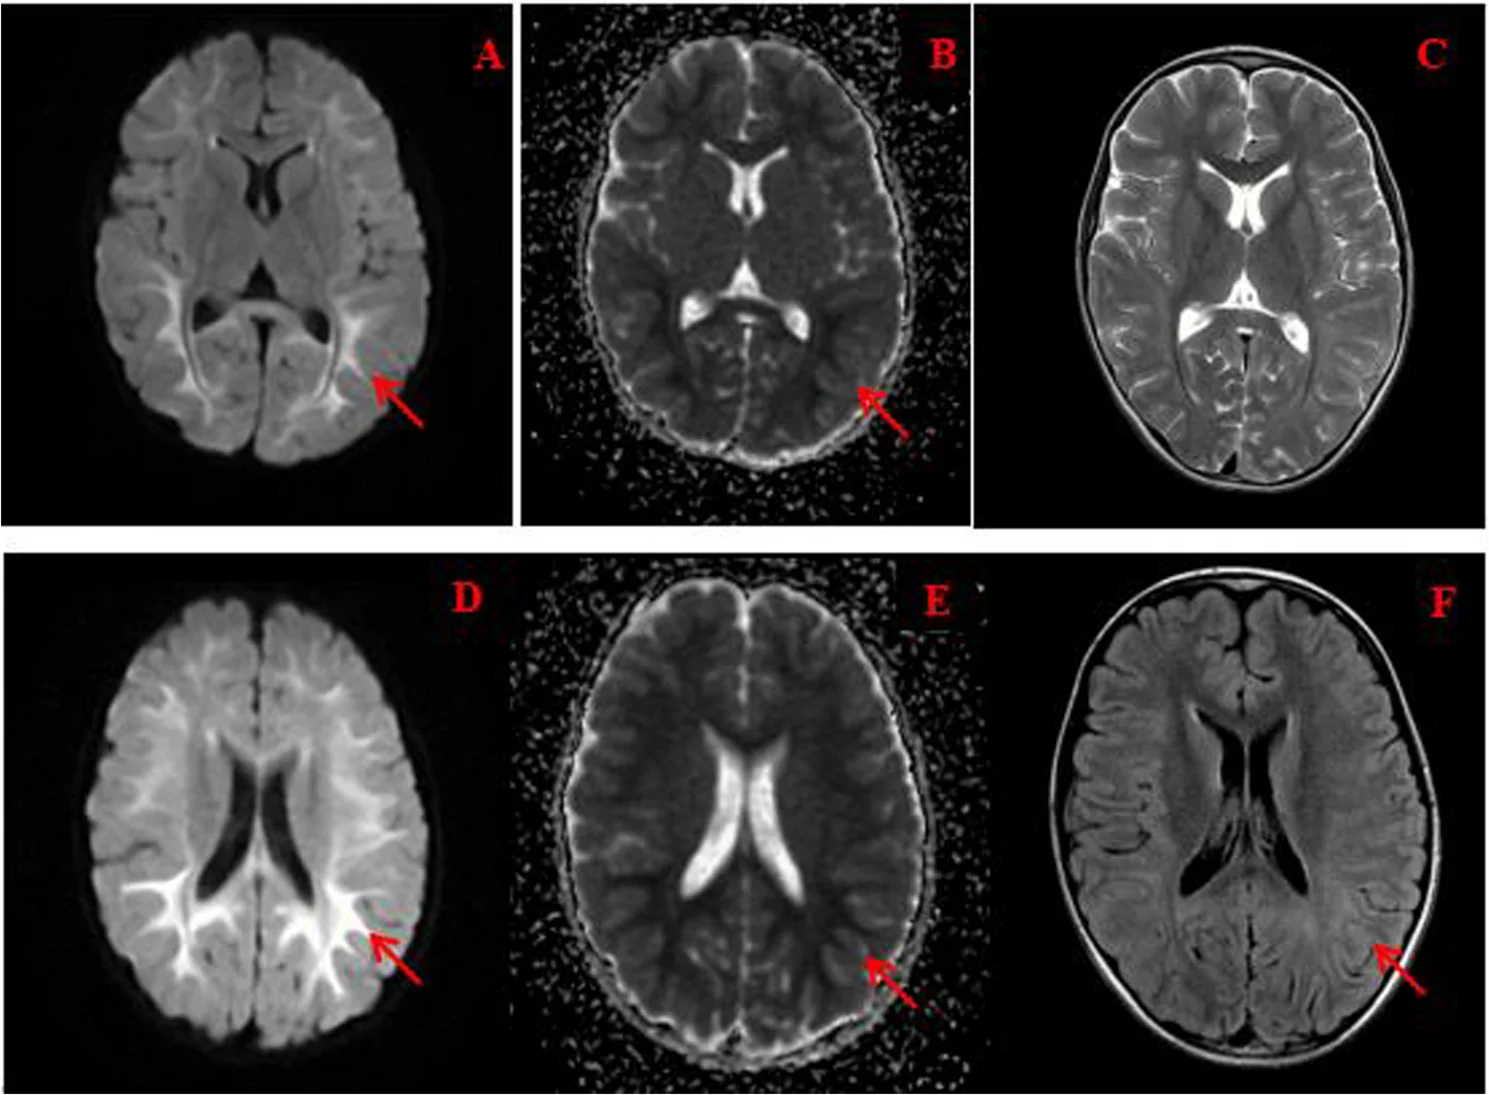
Brain MRI results of children with hypersarcosinemia (April 18, 2025, two and a half months after discharge). A and D: Large patches of DWI hyperintense signal in the subcortical white matter of the bilateral cerebral hemispheres (arrows). B and E: Large patches of subcortical white matter in the bilateral cerebral hemispheres showed hypointense on ADC maps (arrows). C: T2-weighted images showed no abnormalities. F: Slight FLAIR hyperintensity in the white matter of the posterior horn of the bilateral ventricles (arrows). MRI, magnetic resonance imaging. DWI, diffusion-weighted imaging. ADC, apparent diffusion coefficient. FLAIR, fluid-attenuated inversion recovery
